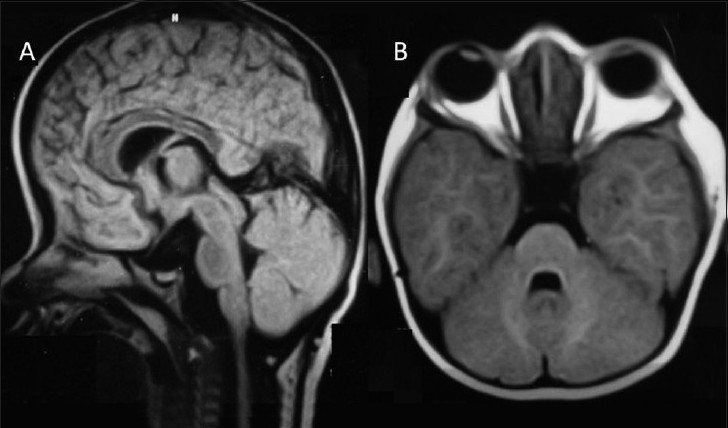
Case 1: (A and B) Magnetic resonance imaging revealed shallow orbits with bilateral proptosis, no Chiari malformation or hydrocephalus
